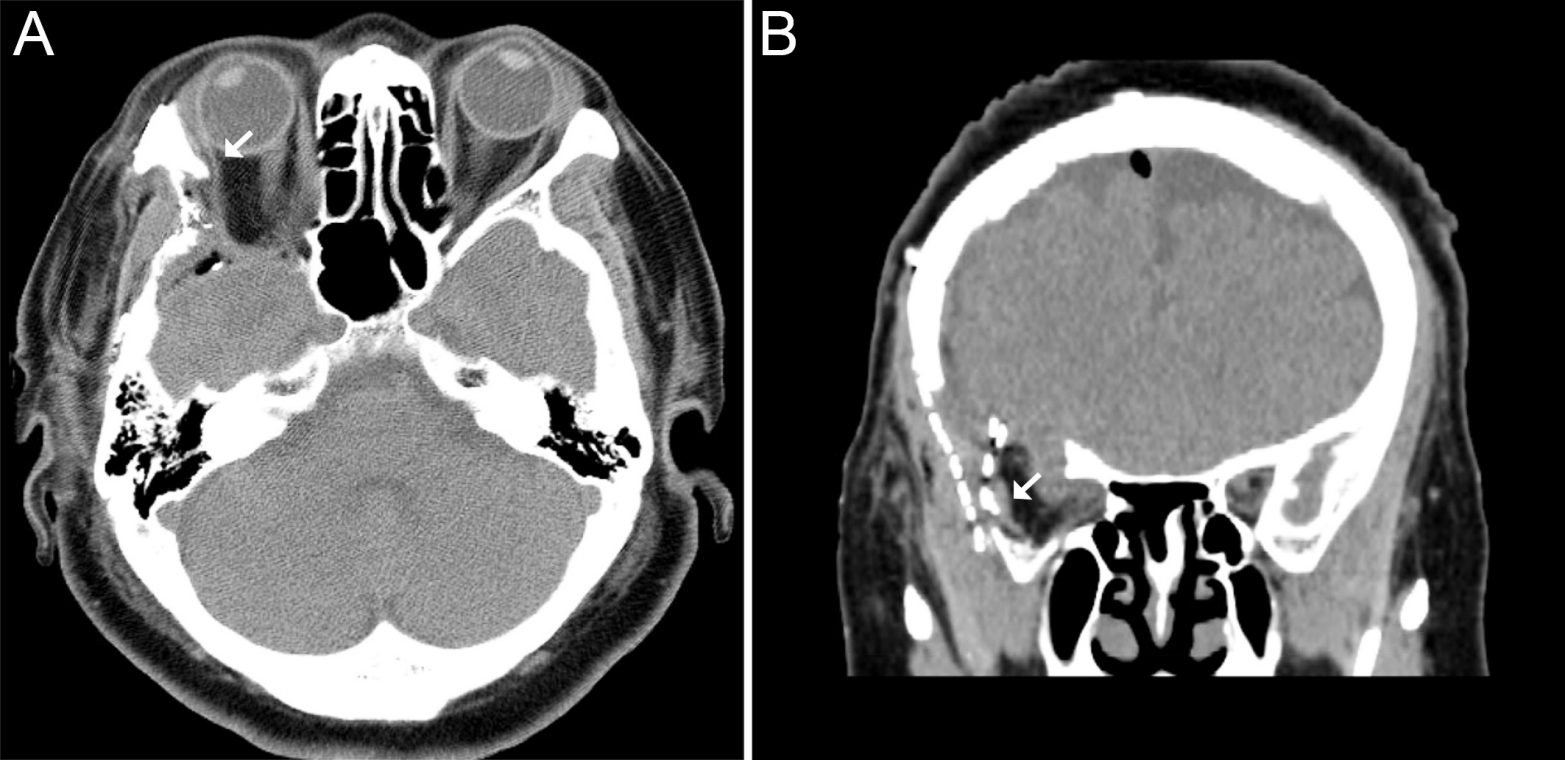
Computed tomography of the orbits in Case 1 Computed tomography of the orbits in Case 1 demonstrates impingement of the right lateral rectus muscle by a residual lip of zygomatic bone on axial image (A, arrow), as well as the alloplastic implant on coronal image (B, arrow).
